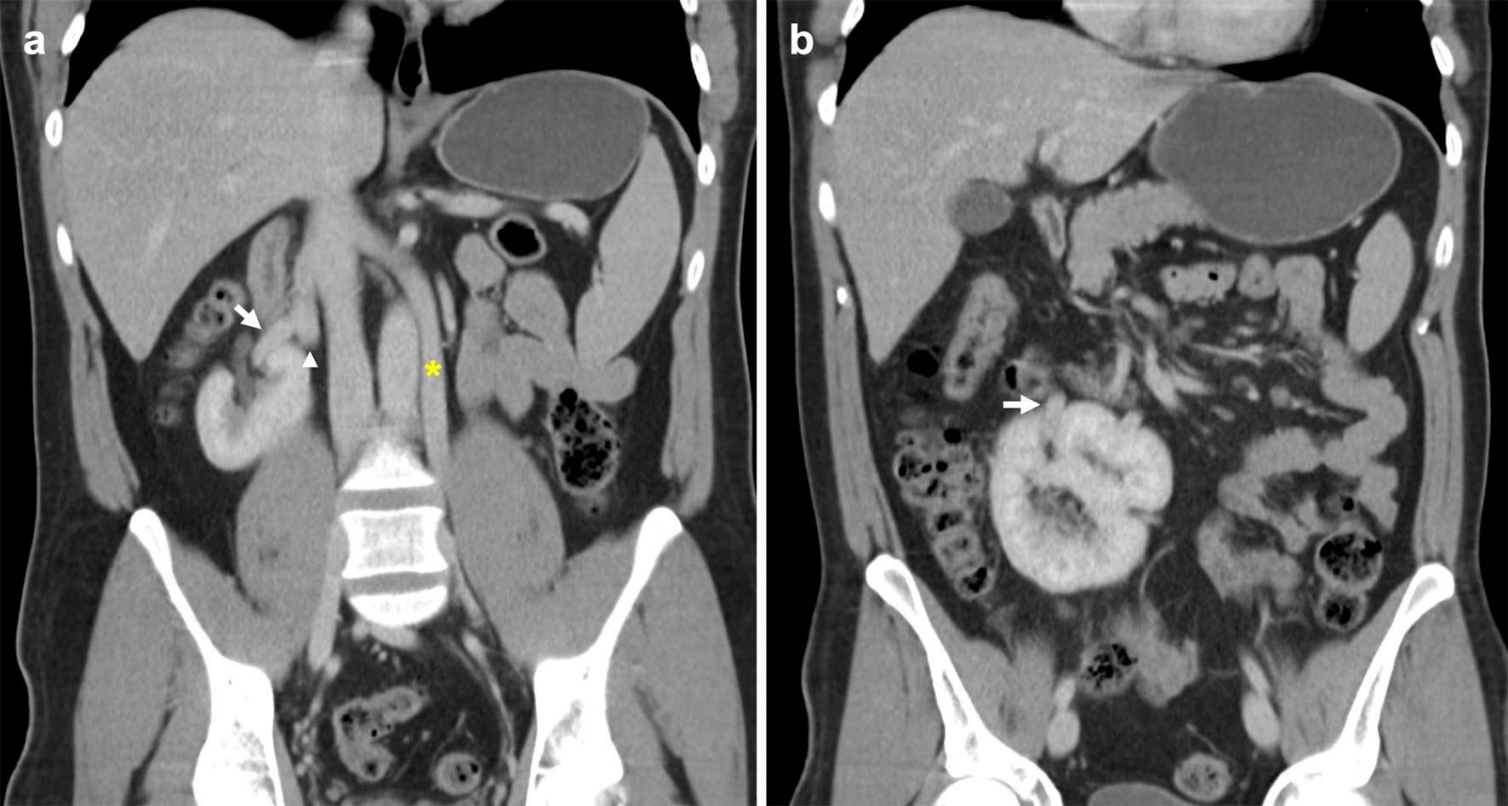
Duplicated IVC with right-sided crossed fused renal ectopia. a Coronal CT image shows a duplicated IVC (asterisk) crossing the midline to drain into the right-sided suprarenal IVC. The right renal vein (arrow) and vein of the left ectopic kidney (arrowhead) join before draining into the orthotopic IVC. b Coronal CT demonstrates the right-sided crossed fused renal ectopia and its renal vein (arrow)
